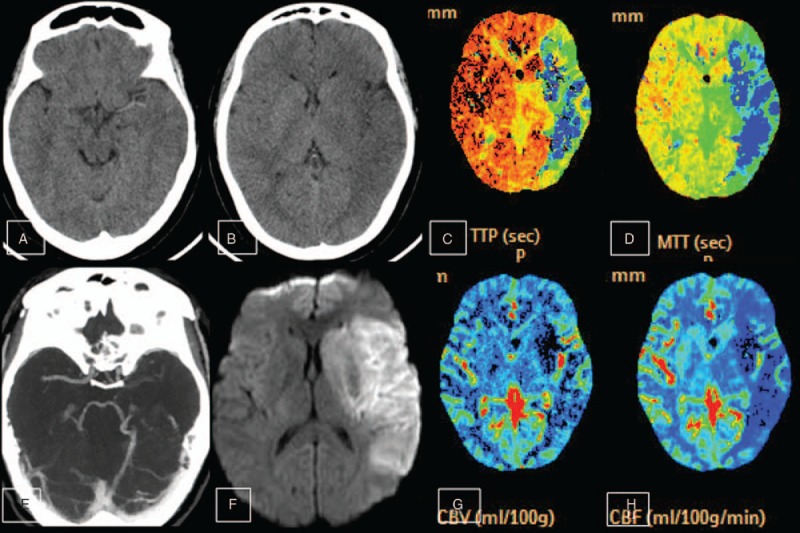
Emergency cranial multiple mode CT and diffusion-weighted images on hospital presentation (day 1). (A, B) Cranial CT showing left middle cerebral artery (MCA) hyperdensity sign with low brain tissue density at admission. (C, D, G, H) CT perfusion showing low perfusion throughout the whole left MCA distribution. (D) Cranial CT angiography (CTA) showing left MCA occlusion. (F) Diffusion-weighted imaging (DWI) showing high signal throughout the MCA distribution, indicating acute cerebral infarction. CT = computed tomography, CTA = CT angiography, DWI = diffusion-weighted imaging, MCA = middle cerebral artery.
